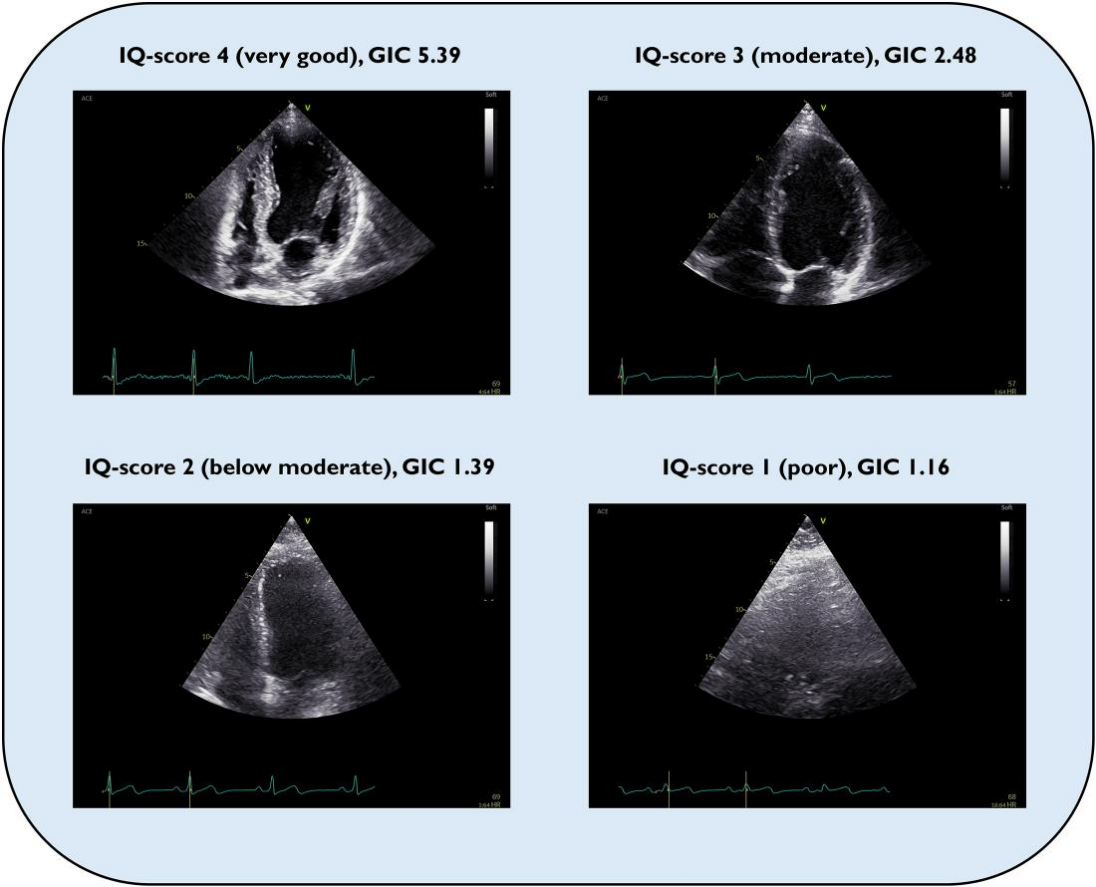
Image quality score and GIC. Image quality-score was rated subjectively from 1 to 4 (4 best) from each cine-loop by two observers. The examples above shows end-diastolic images from LV-focused apical 4-chamber view where both observers agreed on the IQ-score. GIC is an objective measure on the global coherence in the ultrasound image. A low GIC-value is associated with high degree of aberration. The reported GIC is the average value from the complete recording in each view.
